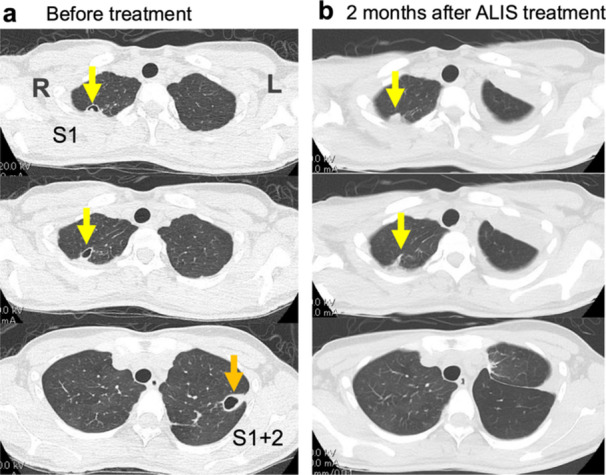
Chest CT images. (a) Pre‐treatment scan showing bilateral cavitary lesions. (b) CT scan 2 months after amikacin liposome inhalation suspension (ALIS) treatment, following segmentectomy of left upper lobe. Yellow arrow: Right upper lobe cavity showing marked alleviation with ALIS therapy. Orange arrow: Left upper lobe cavity.
